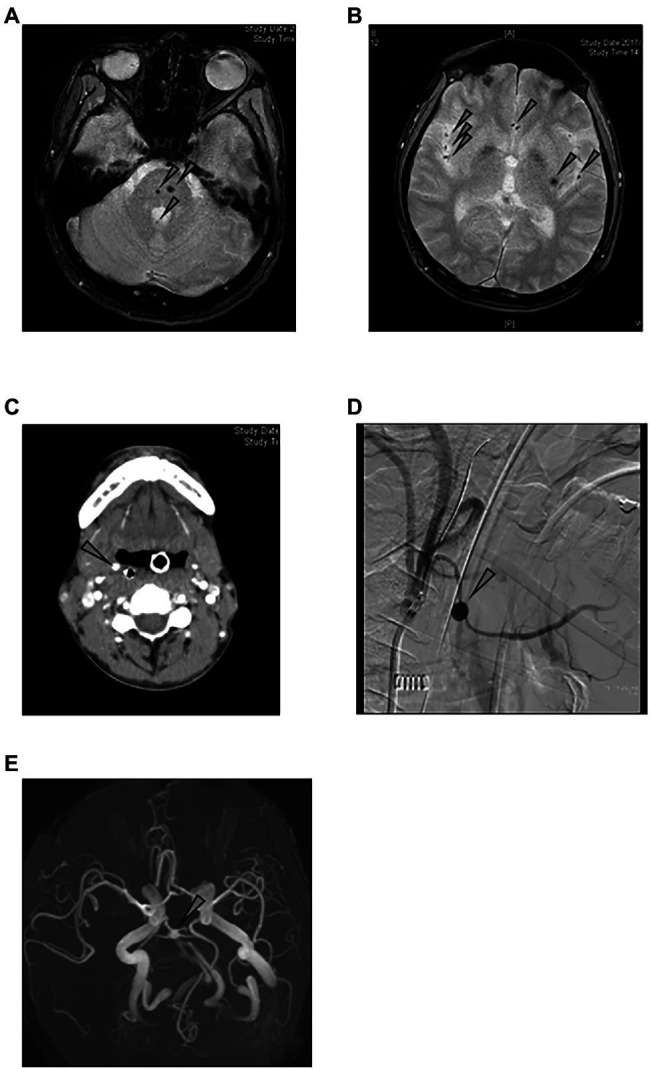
Image findings of sCAEBV patients who had angiopathy. (A,B) Magnetic resonance imaging of the brain of patient 2: Multiple cerebral (A) and cerebellar (B) micro bleeding were detected by T2 star-weighted images (arrows). (C,D) Images of patient 7: Contract-enhanced CT examination shows the dilatation of the right hyoid artery (C). Angiography shows the right hyoid artery aneurysm (D). (E) Magnetic resonance angiography of patient 9: Aneurysm was suspected because of narrowing and dilation of vertebral arteries.
